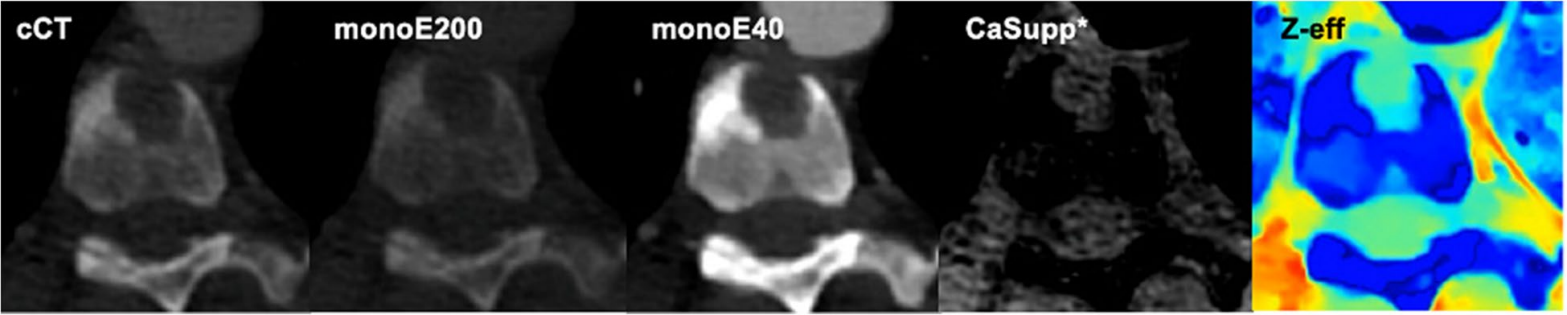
Example of conventional CT and dual-layer CT reconstructions of an osteolytic vertebral metastasis located anteriorly in the vertebral body of Th9 from a 49-year-old female with non-small cell lung cancer. *Abbreviations: cCT, conventional CT; monoE200, monoenergetic 200 keV; monoE40, monoenergetic 40 keV; CaSupp, Calcium suppression; Z-eff, Z effective. *calcium suppression index of 55*
